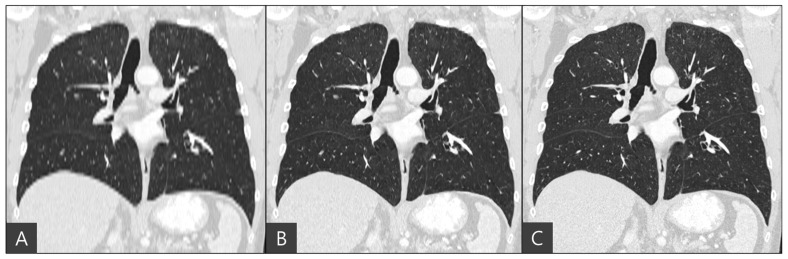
Coronal view of (A) thick-slice, (B) generated thin-slice and (C) thin-slice CT images.
